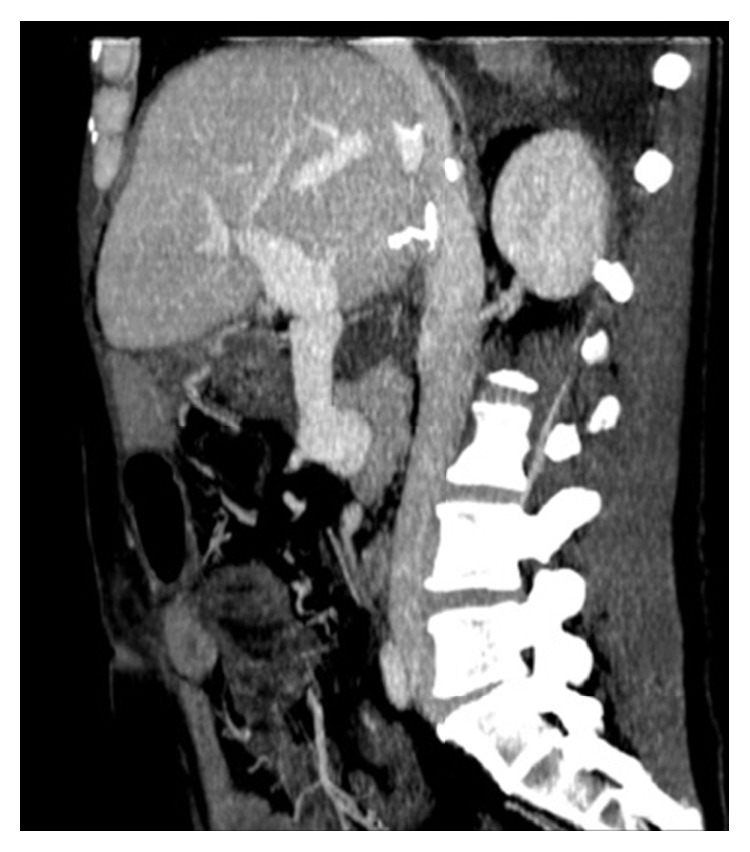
Postoperative CT scan showing regular PV inflow and no anastomotic defects.
